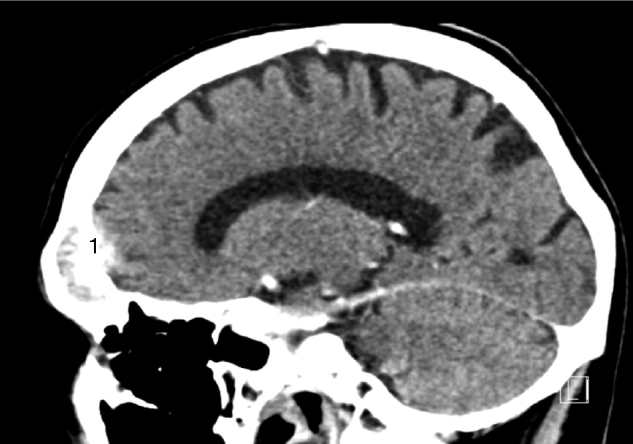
CT images showing a large frontal lesion with bone penetration (1).
